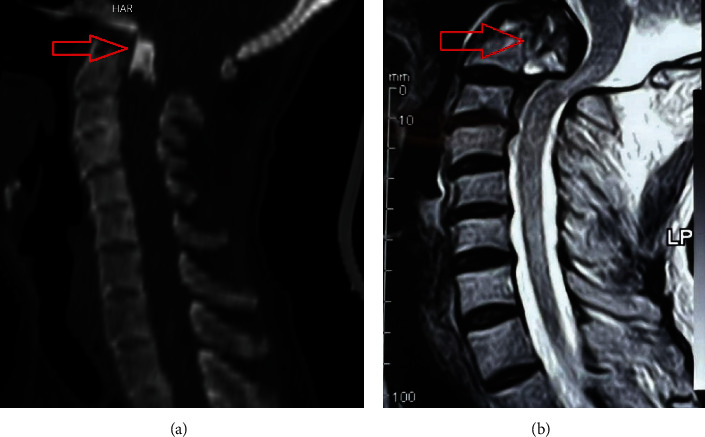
Preoperational midsagittal cervical spinal CT (a) and T2 weighted sequence-MRI (b) showing complete posterior displacement of odontoid process (arrows). CT, computed tomography; MRI, magnetic resonance imaging.
